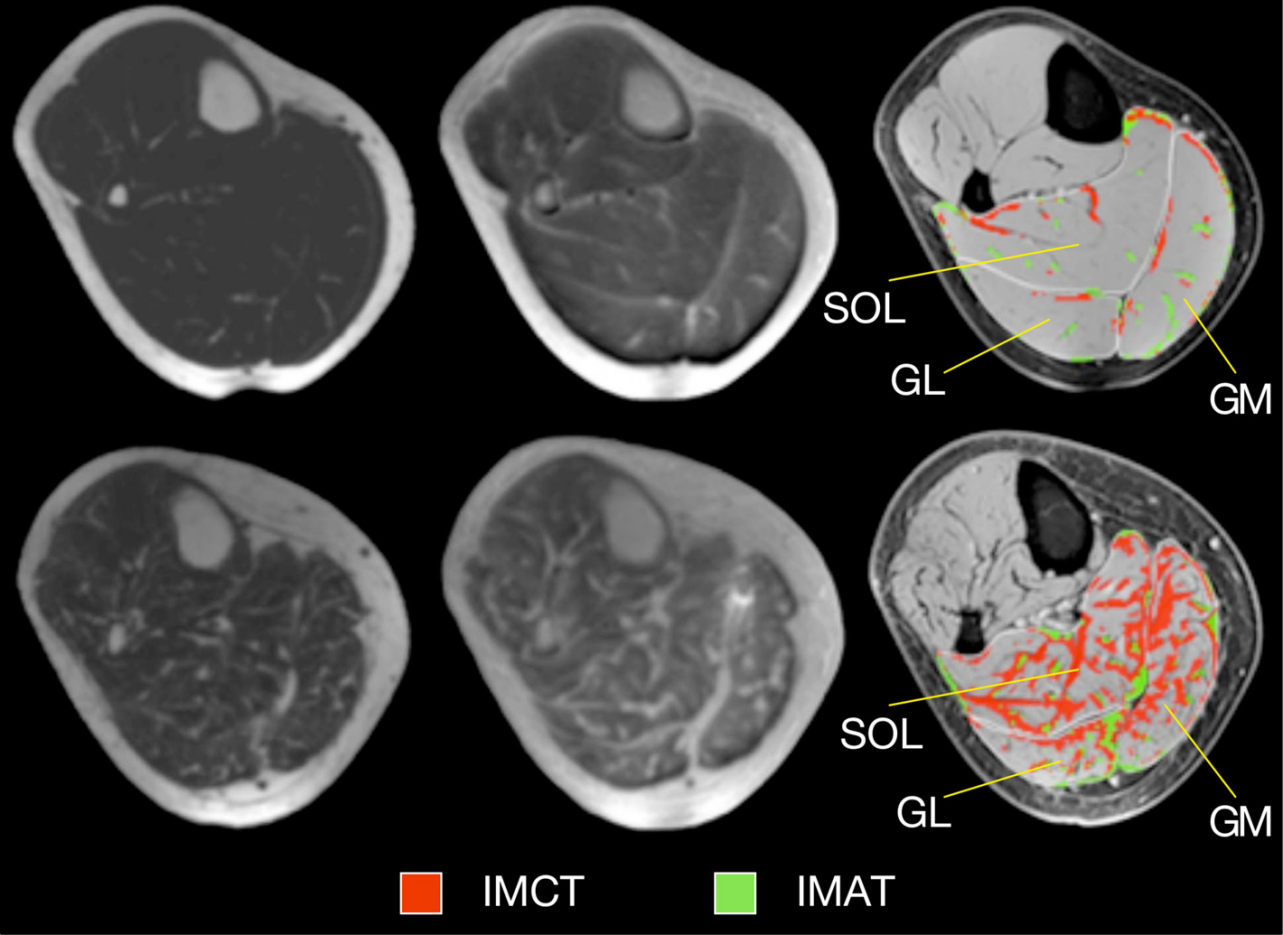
Typical examples of MR images and resulting tissue segmentation in young and older women. Left: Water saturated FGRE (showing IMAT), Middle: UTE (showing IMCT), Right: Standard morphological images with superimposed outer contours of muscles and the result of the automated tissue segmentation. Images in top and bottom row represent one young and old subject, respectively.
